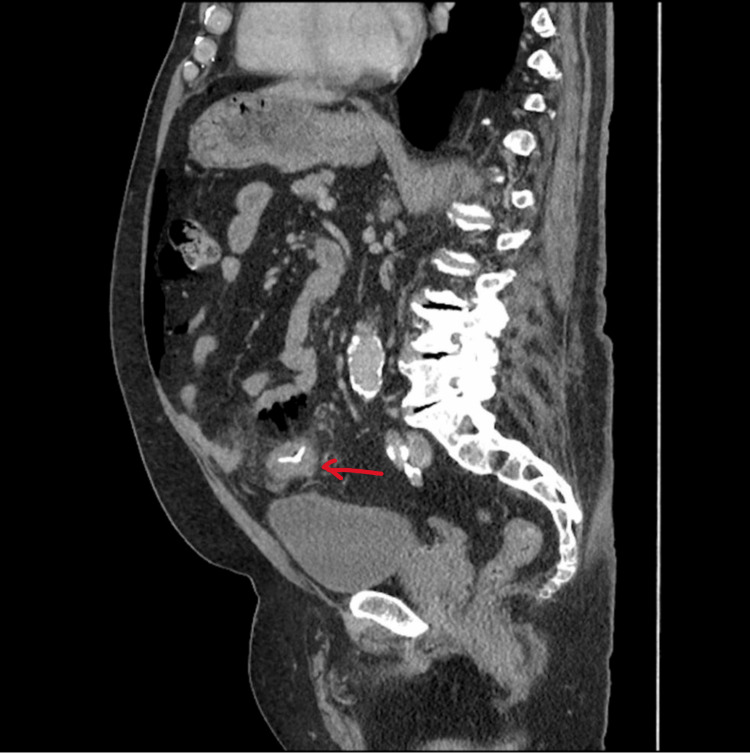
Redemonstration of the ingested wishbone on sagittal contrast-enhanced radiographic imaging at the level of the sigmoid colon, with a proximally thickened bowel segment, new adjacent free air, and a moderate volume of free air throughout the abdomen, concerning for bowel perforation.
